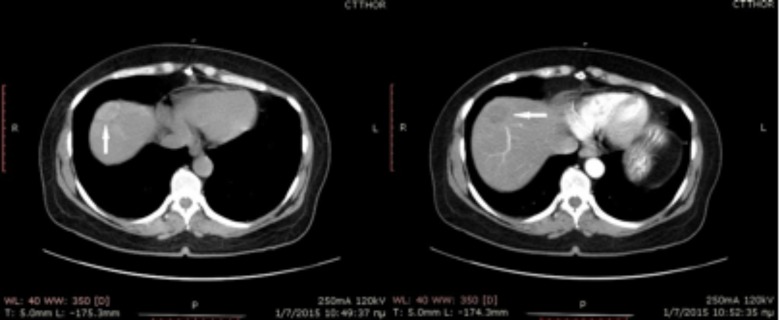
Axial contrast-enhanced CT shows a hypodense lesion at metastasectomy site. The lesion enhances after contrast administration.
